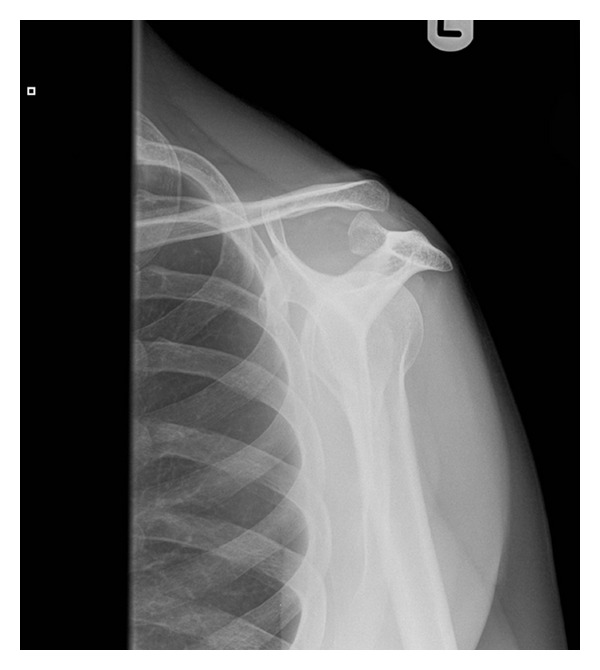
Y view radiograph taken in emergency department at initial presentation.
